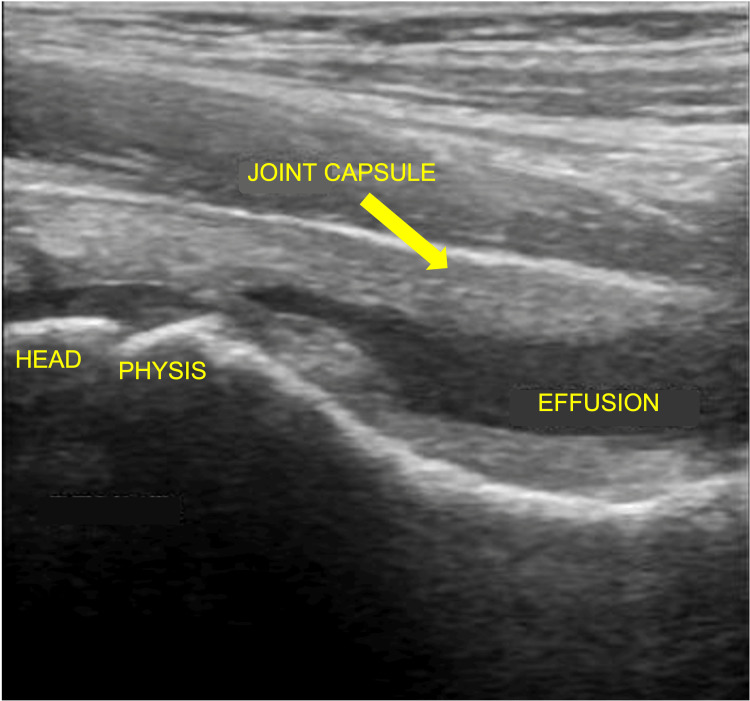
Ultrasound showing joint effusion of the hip Credit: Image adapted from Radiopaedia [25]. Published under Creative Common Licence.
